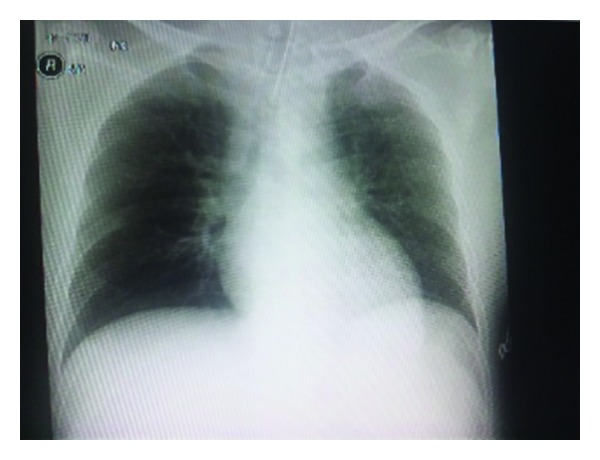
Chest roentgenogram obtained just before extubation in our patient.
